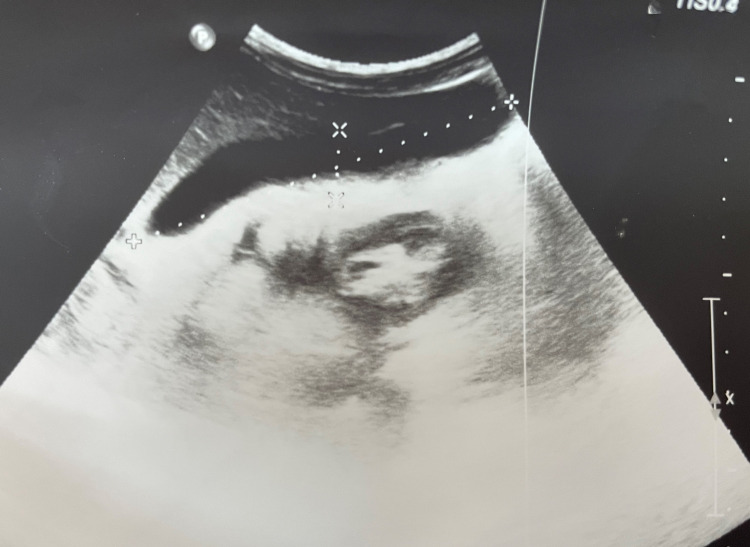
Normal ultrasonography of gallbladder suggesting removal of the worm from the gallbladder
